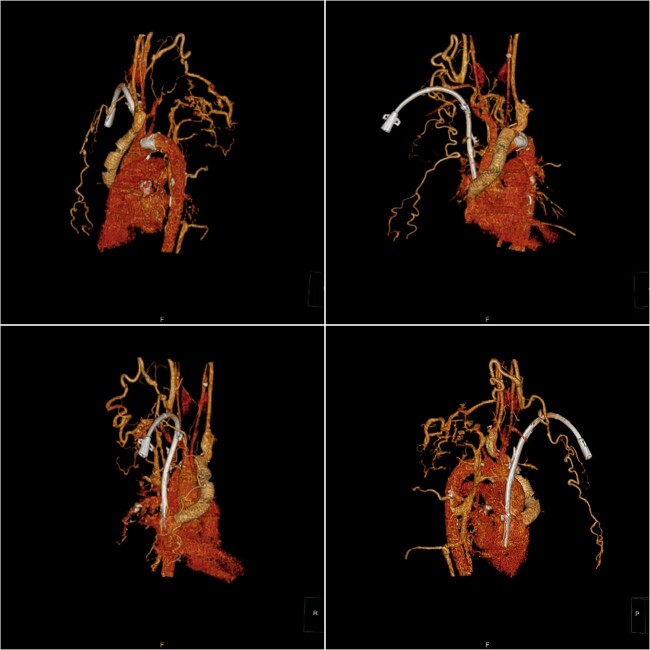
Postoperative computed tomography (CT) three-dimensional reconstruction shows the artificial blood vessel and tunneled cuffed catheter deformation.
